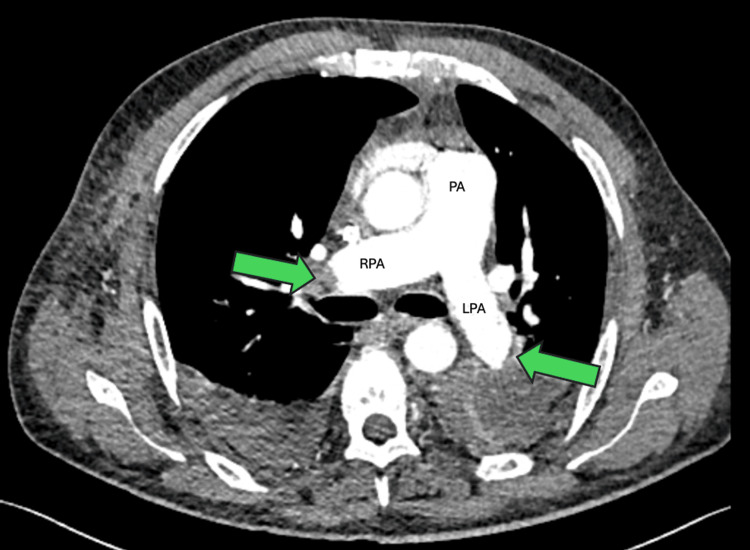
CT pulmonary angiogram (pulmonary arterial phase) showing thrombus occluding the right and left pulmonary arteries (green arrows pointing to the thrombus). PA, main pulmonary artery; RPA, right pulmonary artery; LPA, left pulmonary artery
